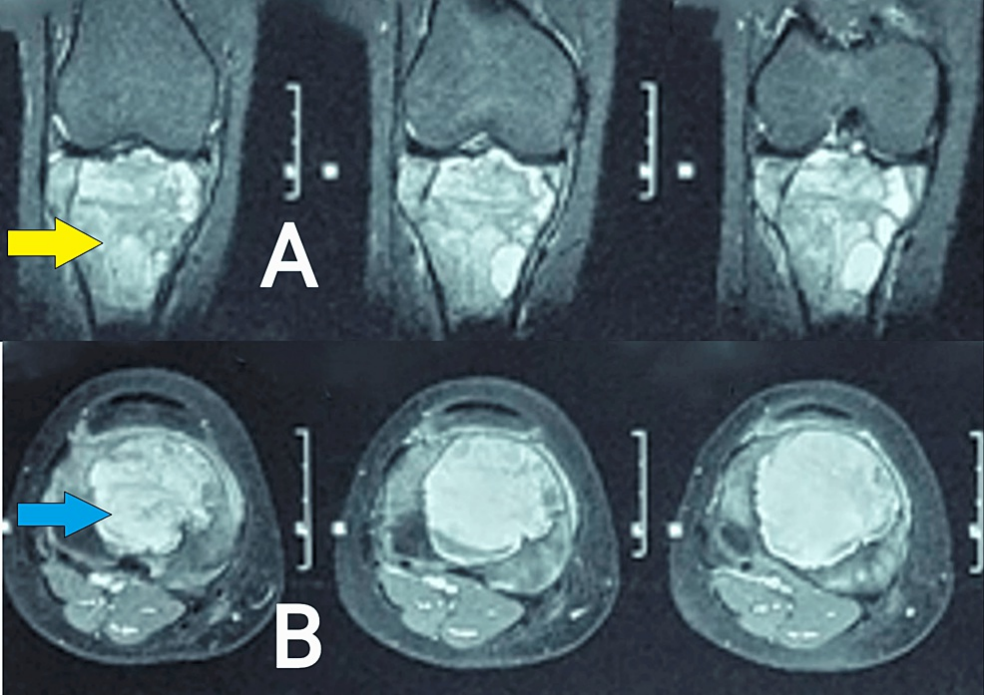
MRI images showing axial and coronal section of the right knee joint with the proximal tibia. (A) Coronal section showing an expansile lytic lesion (yellow arrow); (B) Axial section showing a lytic lesion (blue arrow).
